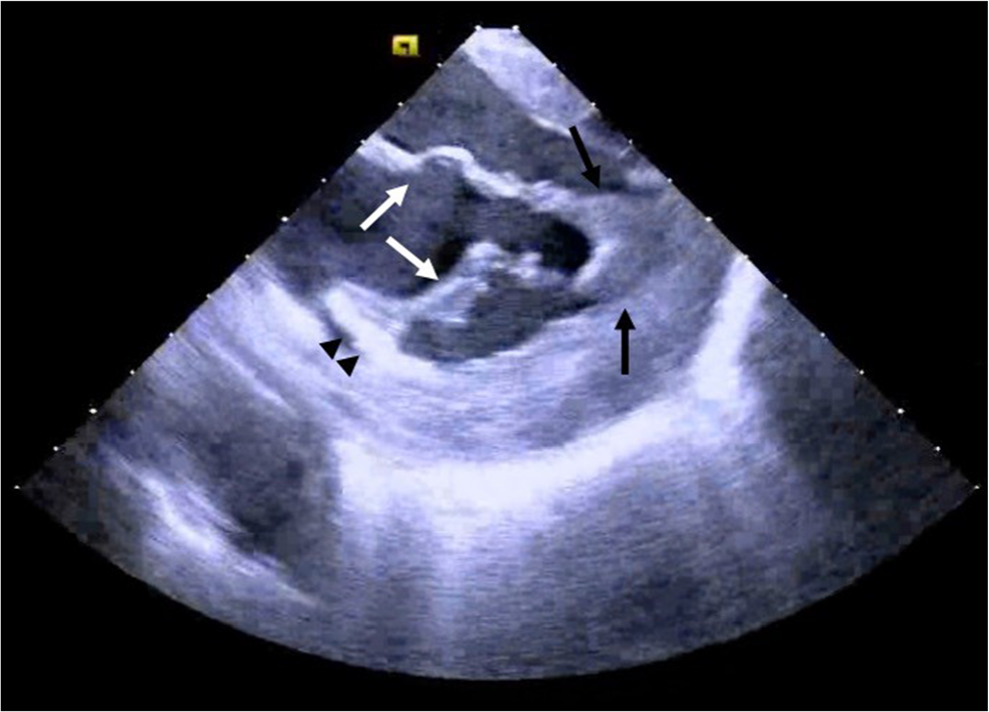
Representative intracardiac echocardiography (ICE) image from ablation of ventricular tachycardia. The black arrows indicate the anterolateral papillary muscle. The white arrows indicate the mitral valve. The black arrowheads indicate the ablation catheter (image courtesy of Ryan Kipp, MD)
